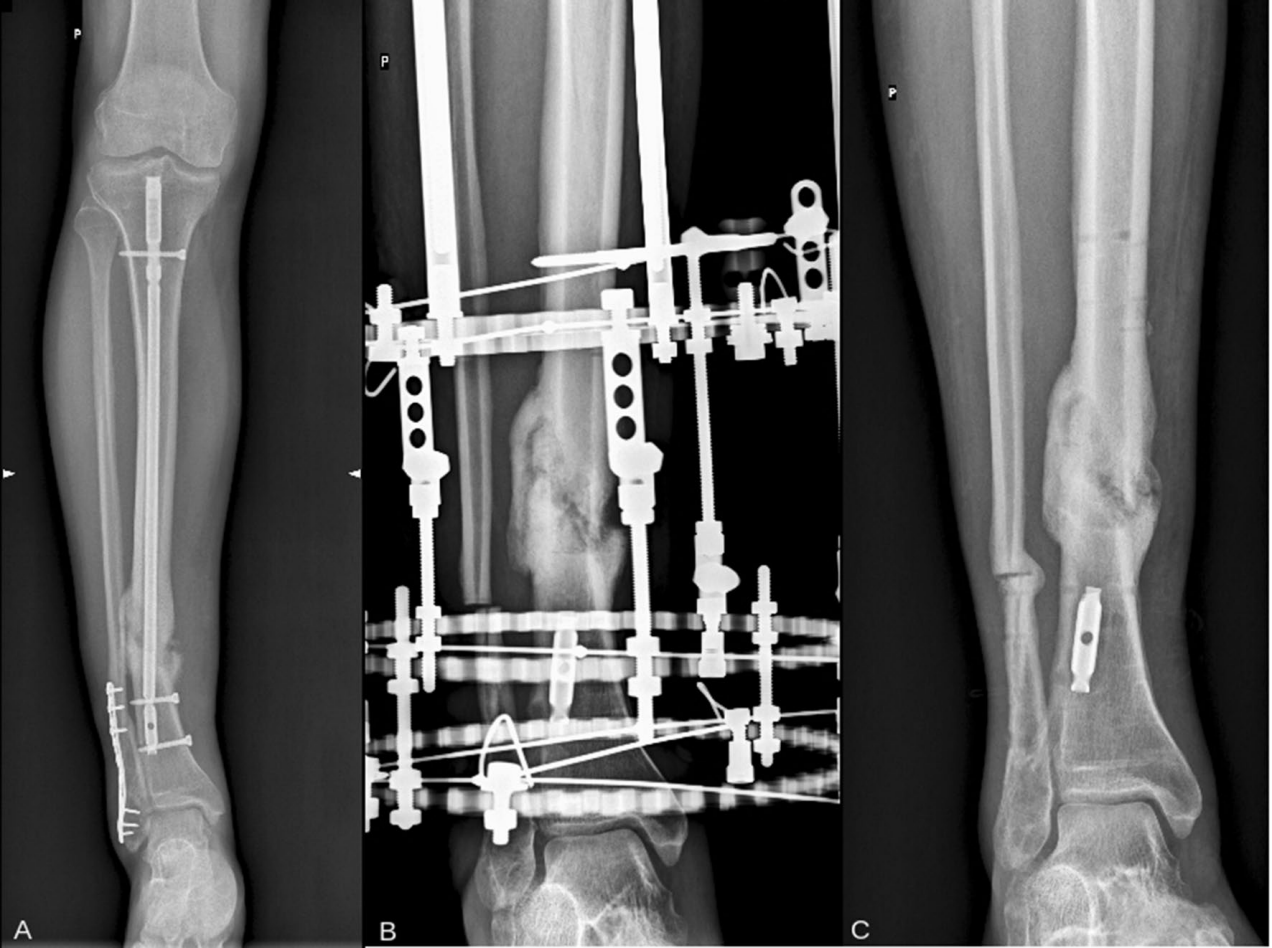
Patient with tibia nonunion. a X-ray from the pre-treatment period, b X-ray with Ilizarov apparatus after union, and c X-ray from the last follow-up visit after 6 years form apparatus removal, confirming maintained union
